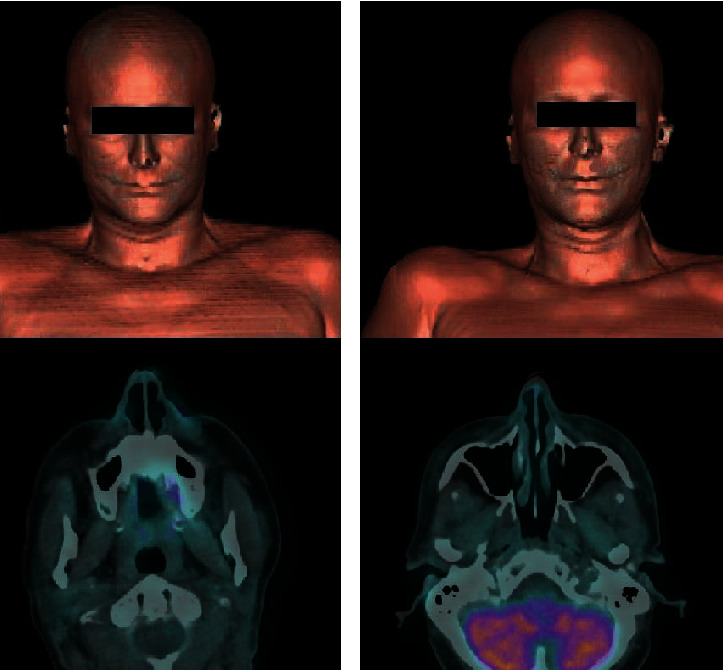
(a) 18F-FDG PET/CT: surface rendering (SR) of the skull (upper row) and corresponding fused axial PET/CT of the zygomatic region (lower row) before filler implant. (b) 18F-FDG PET/CT acquired during follow-up and after filler injection: SR of the skull (upper row) and fused axial PET/CT of the zygomatic region: no abnormal uptake, as a biomarker of inflammation, is evident with respect to the previous scan.
